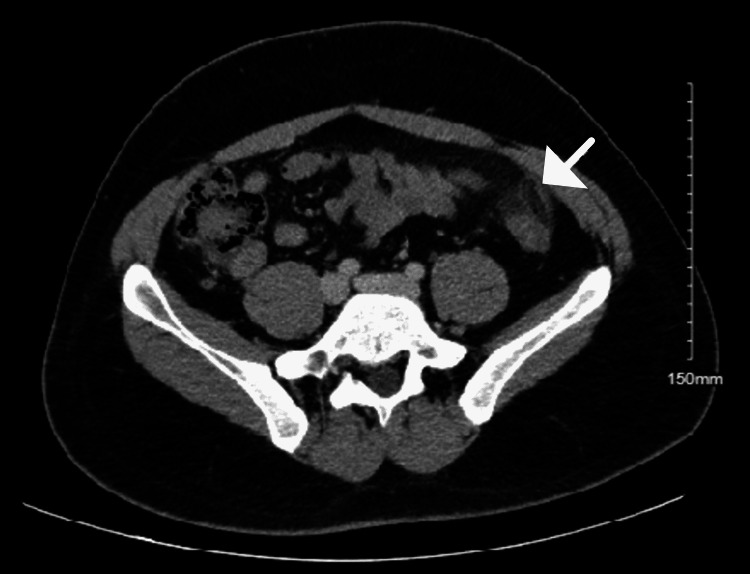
Contrast-enhanced axial section Abdomen and Pelvis CT scan noting epiploic appendagitis at the distal descending colon.
